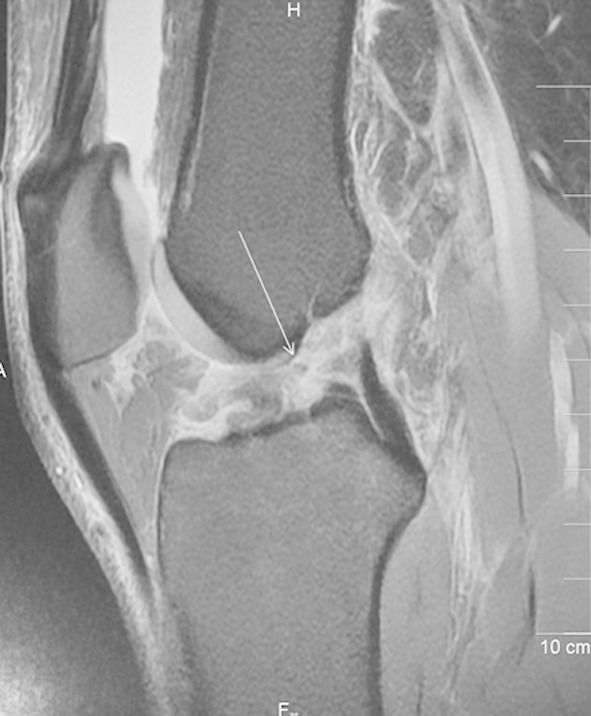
Sagittal TE weighted MR image reveal what was thought to be complete ACL rupture (arrow) was not appreciated as a complete rupture at arthroscopy. According to the arthroscopist it was a partial tear that involved approximately 75% of the ligamentous body.
